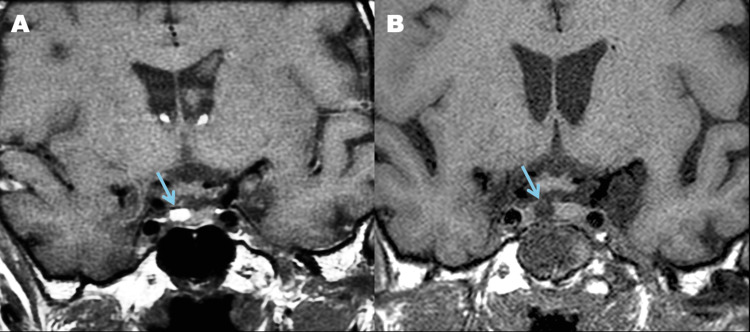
Non-enhanced coronal T1 images before (A) and after (B) procedure. The metastatic lesion exhibited a characteristic high T1 signal (arrow in A). Follow-up MRI showed complete ressection (arrow in B).
